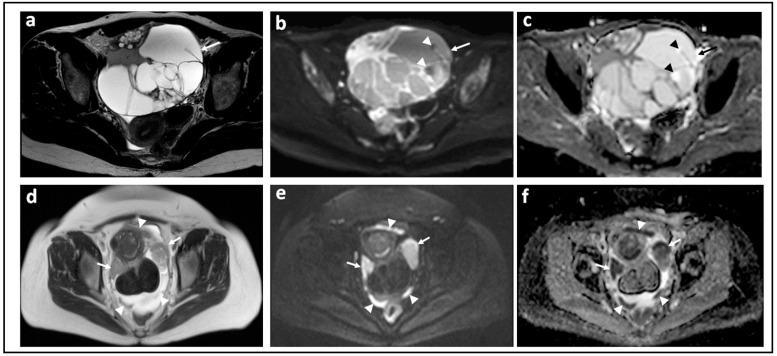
Borderline versus malignant ovarian tumor: T2-W image (a) and corresponding b = 1000 s/mm^2^ DW-MRI (b) and ADC map (c) through the mid-pelvis in a patient with a mucinous borderline tumor of the left ovary. There is a large multiloculated cystic mass with no significant solid components (arrows). Two small foci of low signal in (b) show no evidence of diffusion restriction in c (arrowheads). In comparison, the T2-W image (d), b = 900 s/mm^2^ DW-MRI (e), and ADC map (f) in a patient with invasive high grade serous ovarian cancer show bilateral solid irregular ovarian masses (arrows) and linear peritoneal metastases [arrowheads], all of which show marked diffusion restriction.
